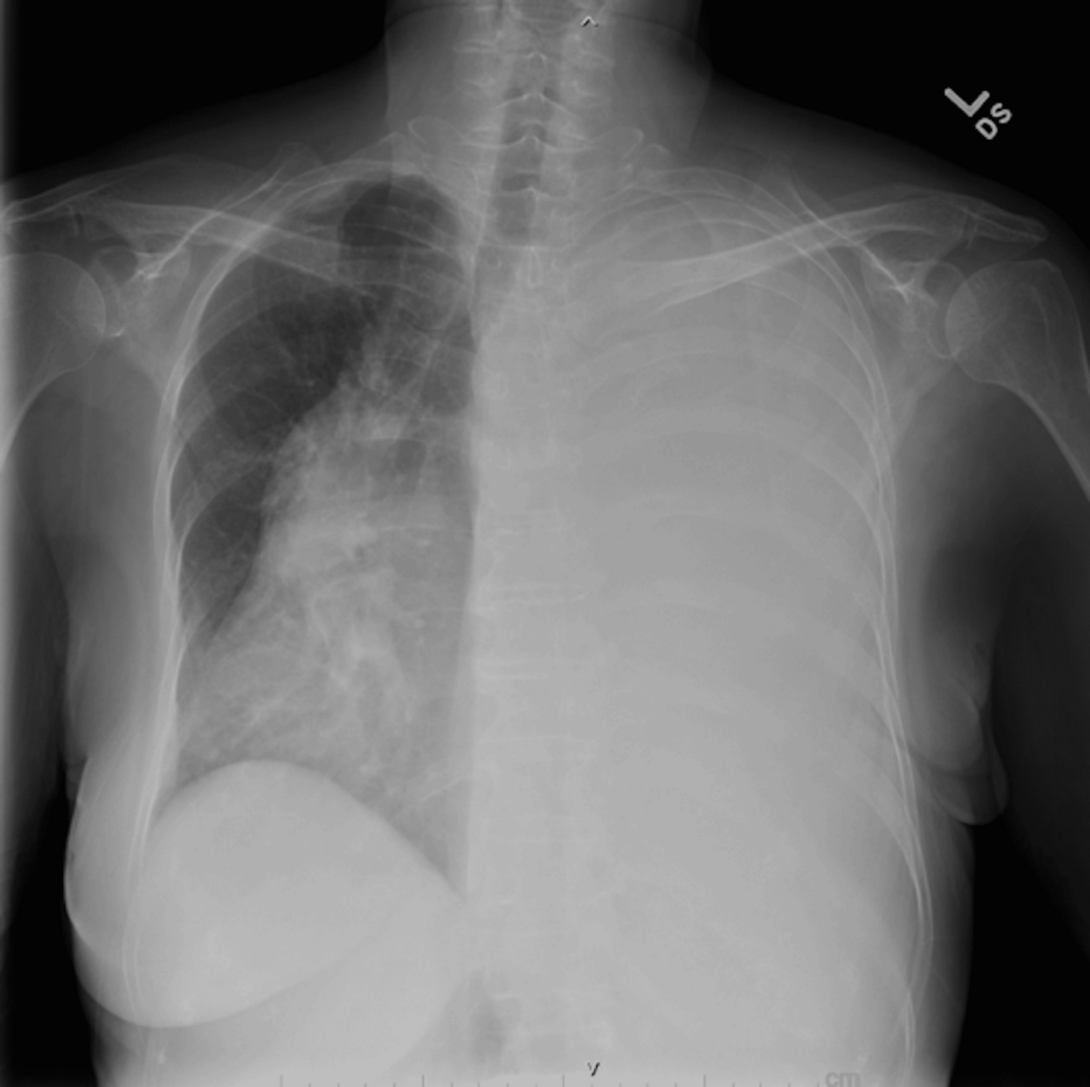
Chest X-ray. Plain radiograph of the chest showing opacification of the left hemithorax, with dextroposition of the heart and mediastinal structures to the right.
